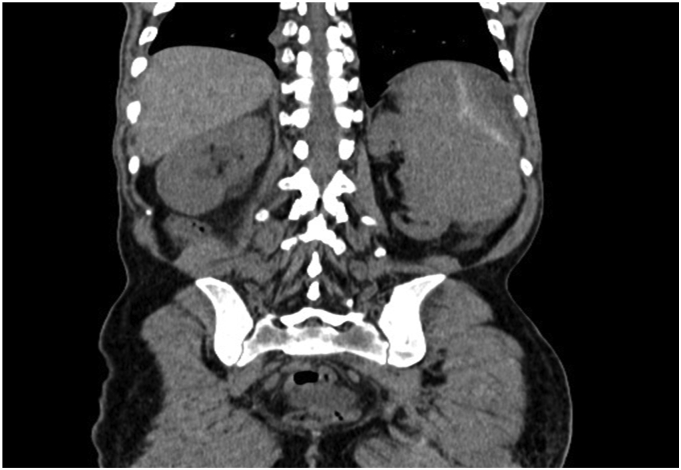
CT image showing splenomegaly with subcapsular hematoma of the spleen.
